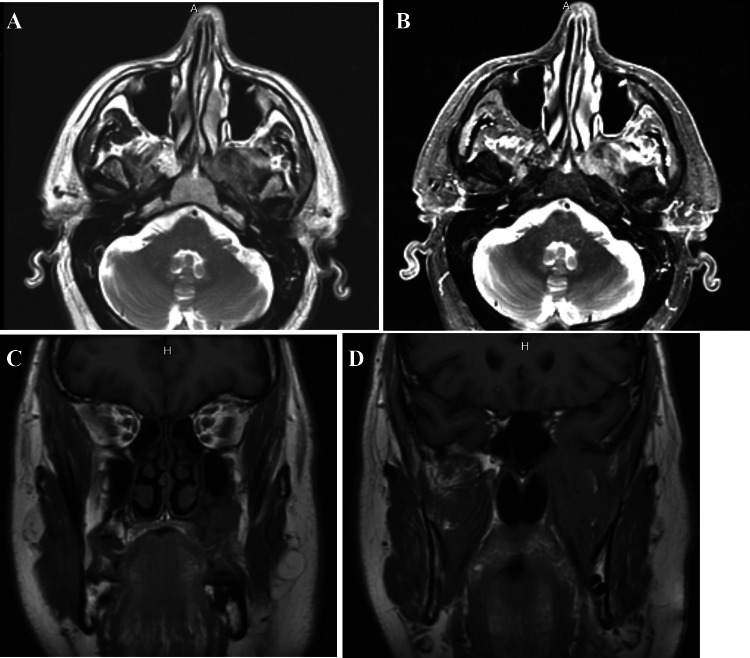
A–D: T1-weighted axial (A), T2-weighted axial (B), and T1-weighted coronal (C, D) MRI images. Indicating a soft tissue mass with hypointense signal intensity on T1-weighted and heterogeneous signal intensity on T2-weighted images, affecting the left posterior alveolar bone of the maxilla, the left infratemporal fossa, base of the skull, pterygopalatine fossa, and sphenopalatine foramen
